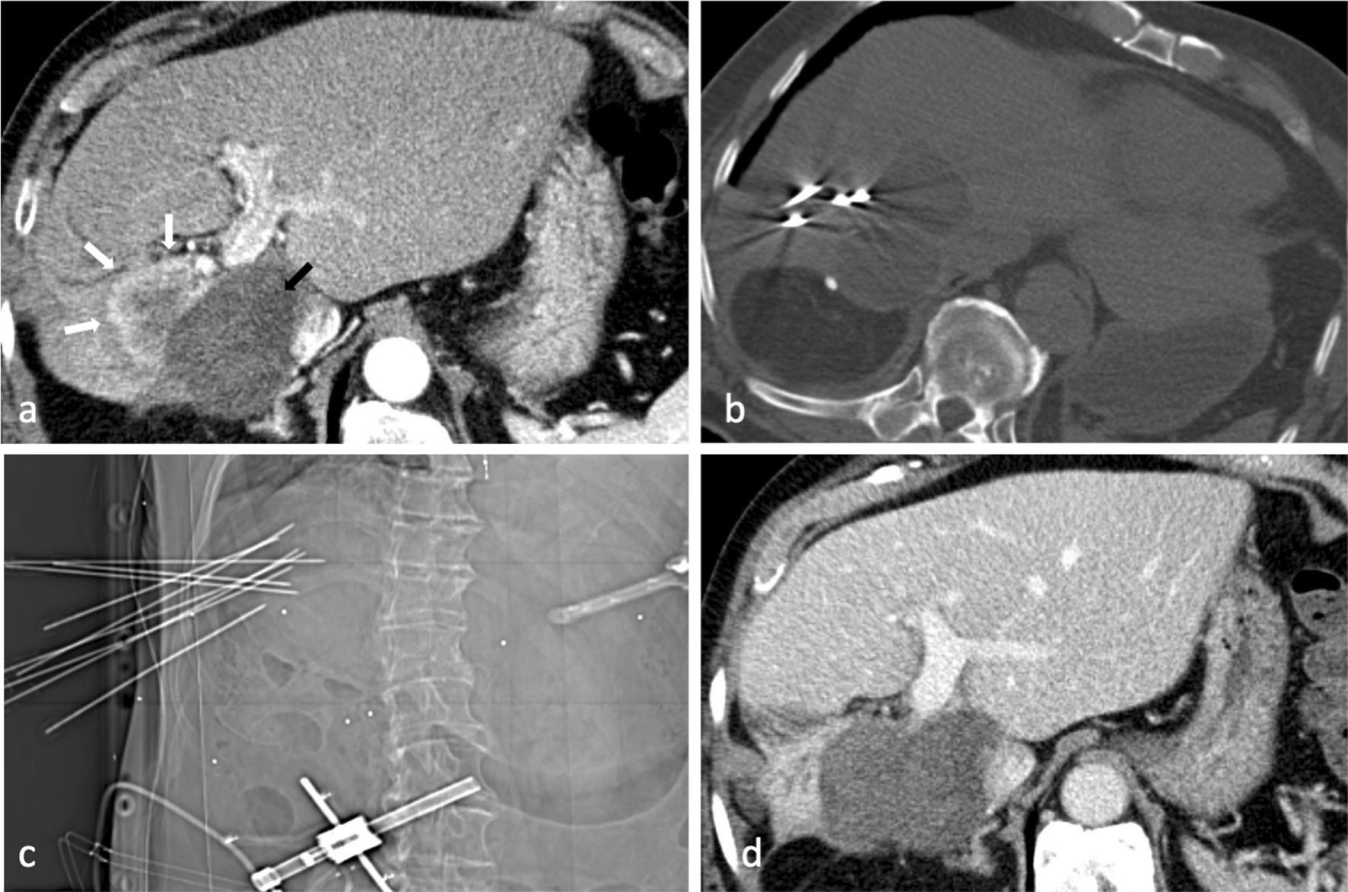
A Arterial phase CT showing local recurrence with 4.5 cm in diameter (white arrow) directly adjacent to large necrosis zone (black arrow) in November 2012. B, C Axial non-enhanced control CT and scout view, (C) with nine coaxial needles in place during the fourth SRFA session in December 2012. D First follow-up with no evidence of residual/recurrent tumor 4 months later (March 2013)
